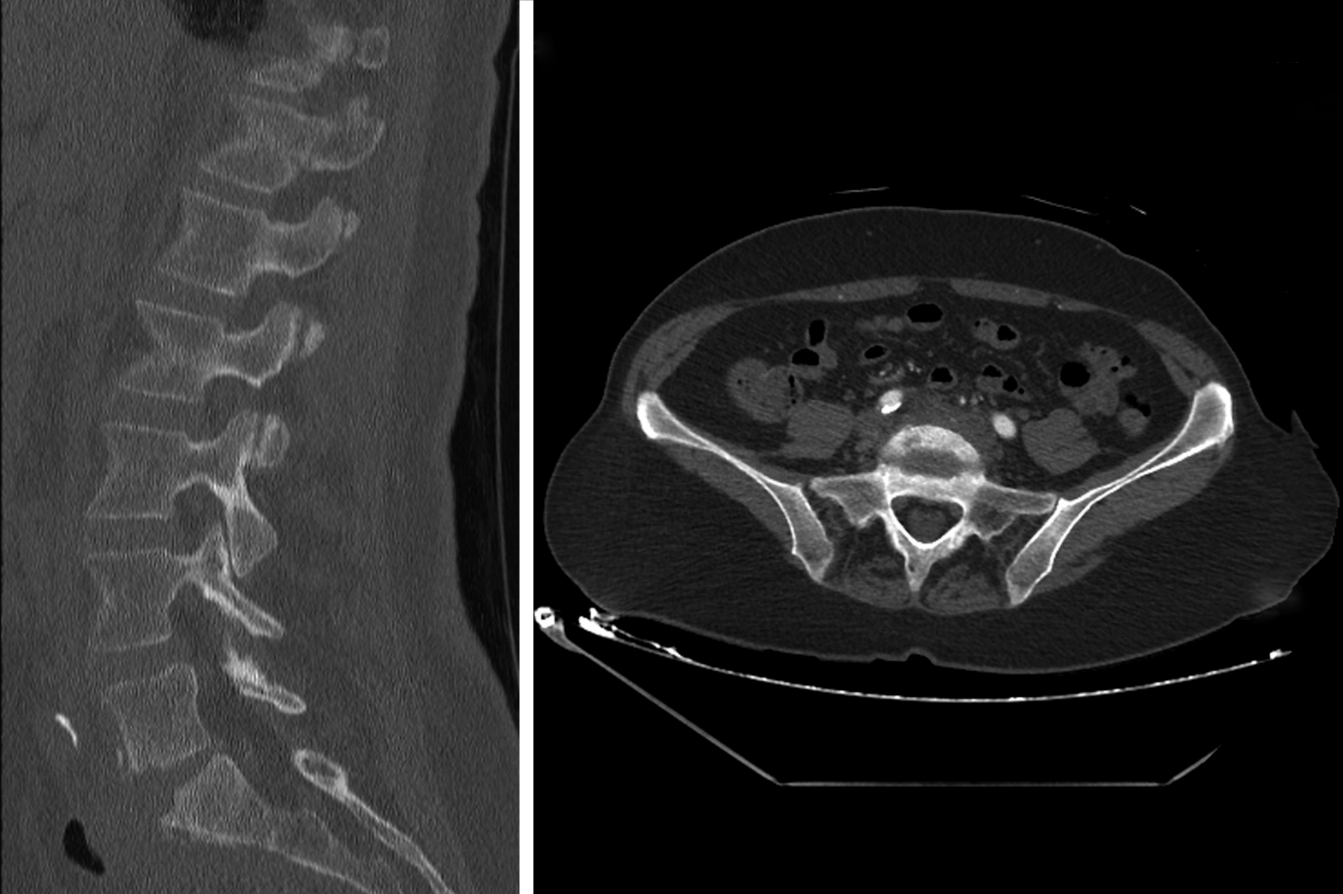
CT and CTA of Lumbar Spine Figure 2: Sagittal CT of lumbar spine with endplate destruction at L5 and S1 (left) and axial CTA pelvis (right) with iliac arteries embedded in soft tissue mass.
